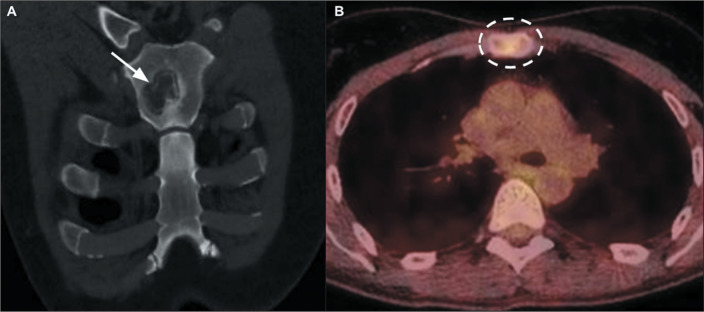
Osteomyelitis of the sternum. A: Coronal CT scan of the chest, with bone
